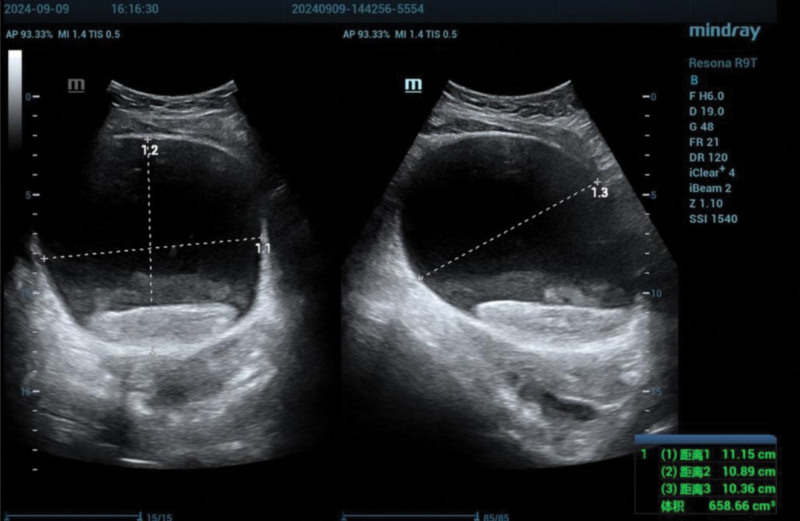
Ultrasonography demonstrating severe bladder distension. AP = anteroposterior, DR = dynamic range, FR = frame rate, MI = mechanical index, SSI = spatial standard intensity, TIS = thermal index scan.
